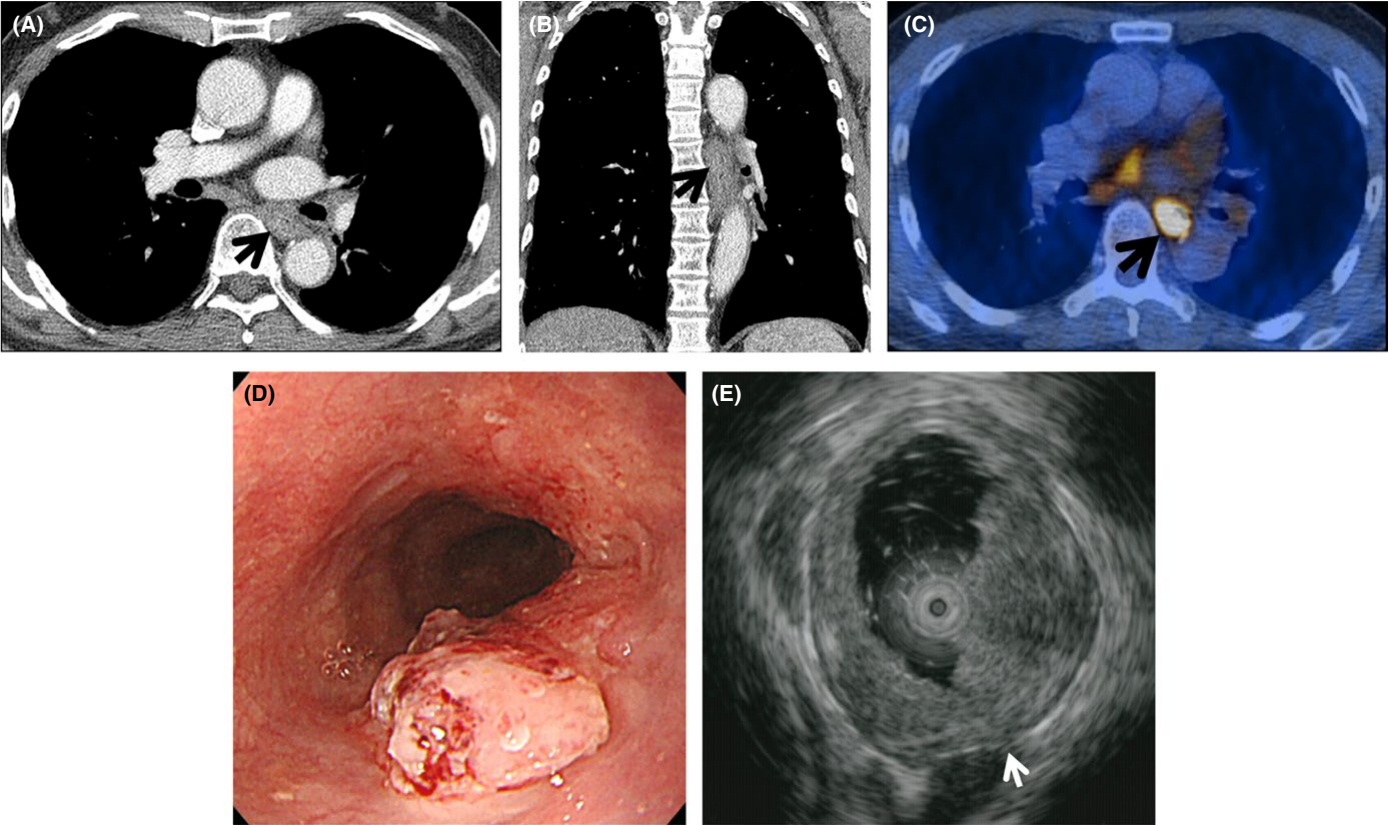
pT2N1‐stage esophageal squamous cell carcinoma in a 74‐year‐old man involving the intrathoracic middle esophagus. (A, B) Transverse (A) and coronal (B)‐reformatted CT images show circumferential wall thickening in intrathoracic middle (subcarinal) esophagus with its posterior wall thickness of 10.5 mm. (C) PET/CT demonstrates hypermetabolic lesion at the area of CT abnormality with its SUVmax, 18.5. (D) Endoscopy depicts a 3‐cm‐sized semi‐circumferential protruding tumor with surface nodularity in the intrathoracic middle esophagus. (E) Tumor is infiltrating into the proper muscle layer (arrow) on EUS. On pathologic examination, G3 (left gastric) node was positive for cancer cells; either EUS or PET/CT failed to detect the node
